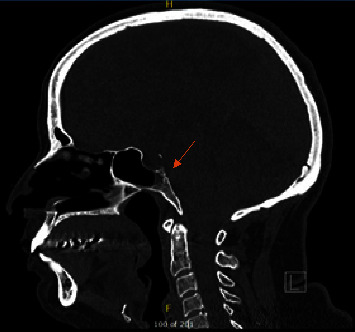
CT sinus without contrast sagittal cut showing the air-fluid level in the sphenoid sinus and defect in the clivus (see arrow).
